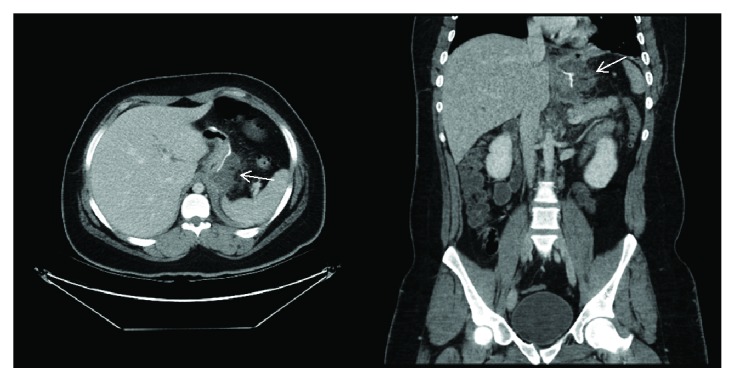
Computed tomographic scan showing perigastric collection adjacent to gastroesophageal junction and along sleeve gastrectomy sutures. Arrow: perigastric collection.
